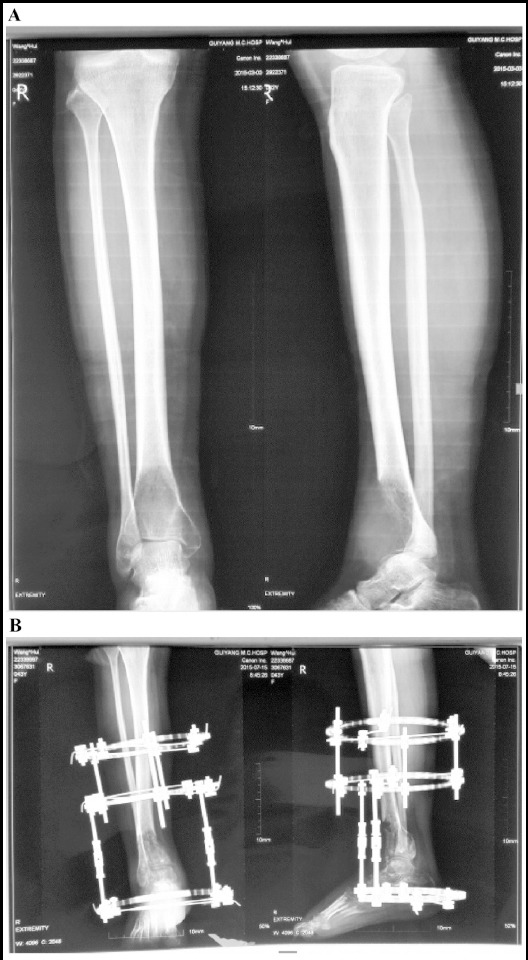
Representative X-ray films before (A) and after (B) TSF fixation.
